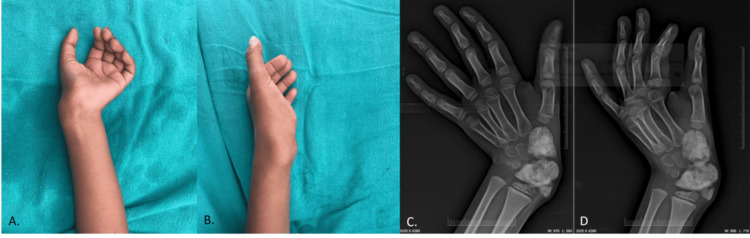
(A, B) Clinical images showing the swelling on the lateral and volar aspects of the left wrist. (C, D) X-ray AP and oblique views demonstrate an expansile sclerotic lesion involving the scaphoid, lunate, trapezium, trapezoid, and the inferolateral aspect of the scaphoid, with the capitate, hamate, and triquetral bones appearing normal.
